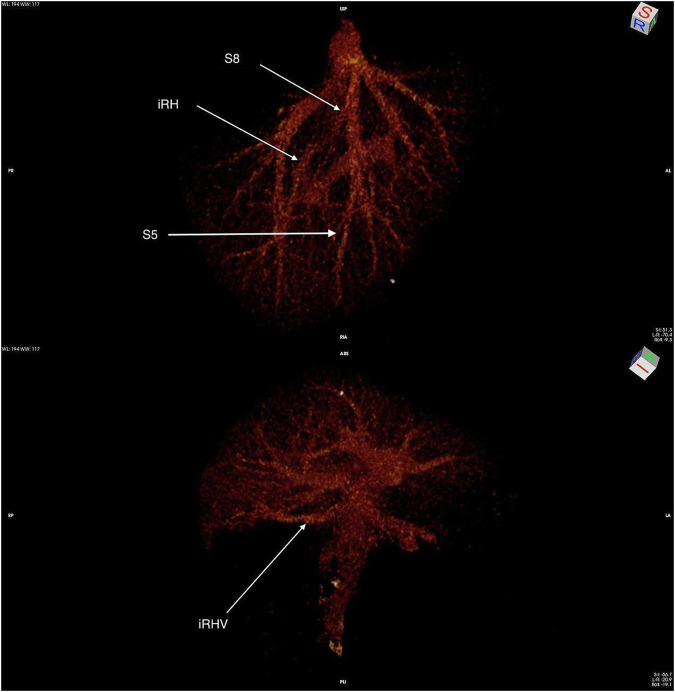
Three-dimensional CT reconstruction of the donor’s venous outflow. Arrows indicate the significant venous branches of segments 5 and 8, as well as the inferior right hepatic vein (IRHV).
